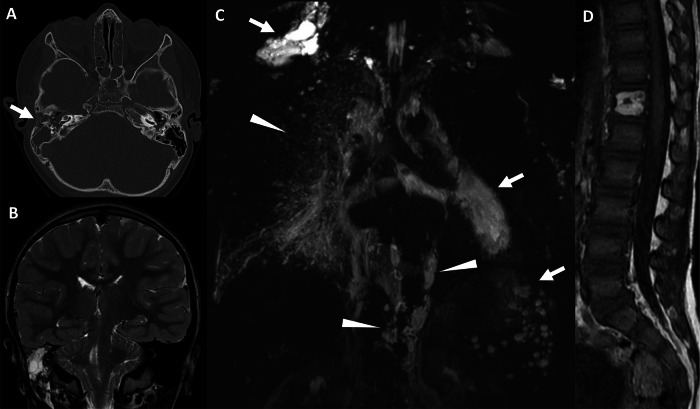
Recurrent meningitis in a 4-year-old boy revealing GLA with bone destruction (Gorham–Stout disease). A Noncontrast CT, axial view. Osteolysis of the right petrous apex with osteomeningeal fistula (arrow). B T2w, coronal view. Lobulated lesion of the right petrous apex with high signal intensity. C 3D FRFSE T2w, MIP reconstruction (20 mm), coronal view. NEMRL reveals diffuse lymphatic anomalies: supra-clavicular, mediastinal, and splenic lymphangiomas (arrows) associated with peribronchovascular and paravertebral lymphangiectasis (arrowheads). D T1w, sagittal view. Fat-containing amorphous lesion of the Th12 vertebral body with high signal intensity
